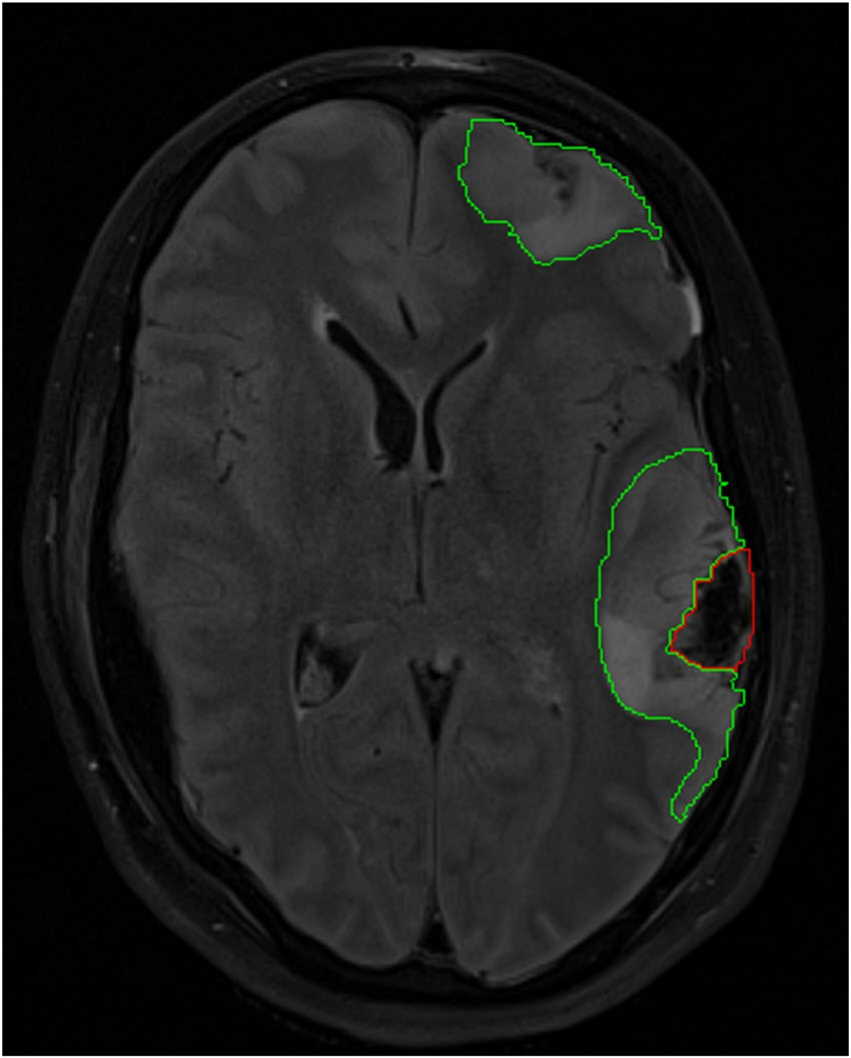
Lesion regions of Interest. Fluid attenuated inversion recovery (FLAIR) image from subject 8 with lesion core and contusion highlighted on a single axial slice.
